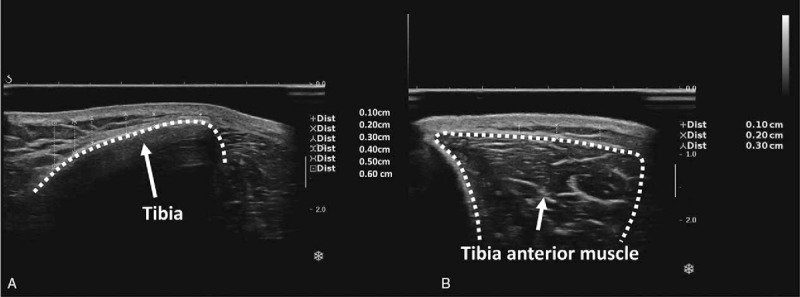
(A) and (B) are the sonograms when assessing the skin above anterior and lateral tibia. The thickness of subcutaneous fat above tibia and tibialis anterior muscle showed a graded increasing trend.
